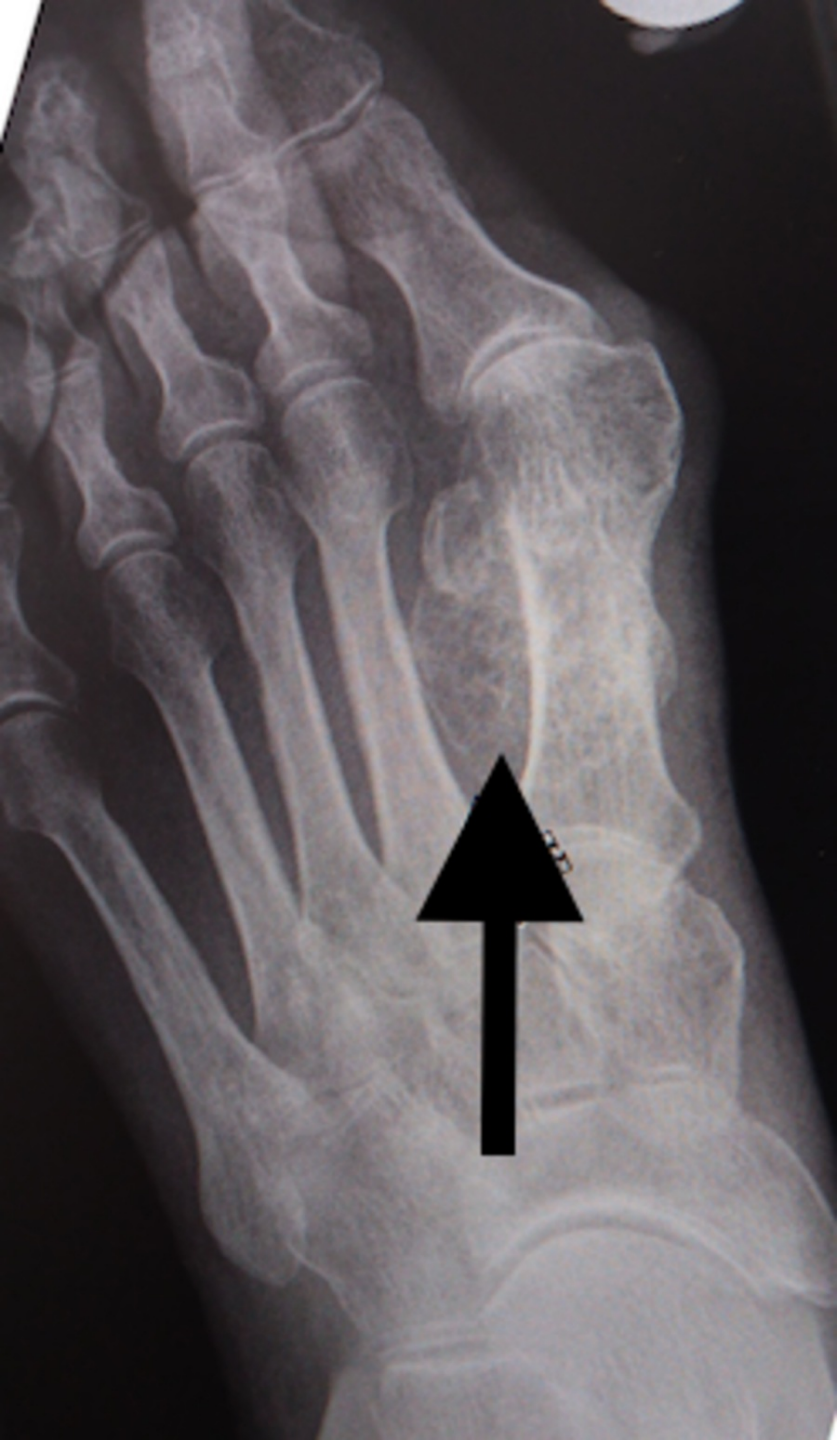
X-ray of osteochondroma. Arrow showing the osteochondroma on plantar surface of 1st metatarsal.
